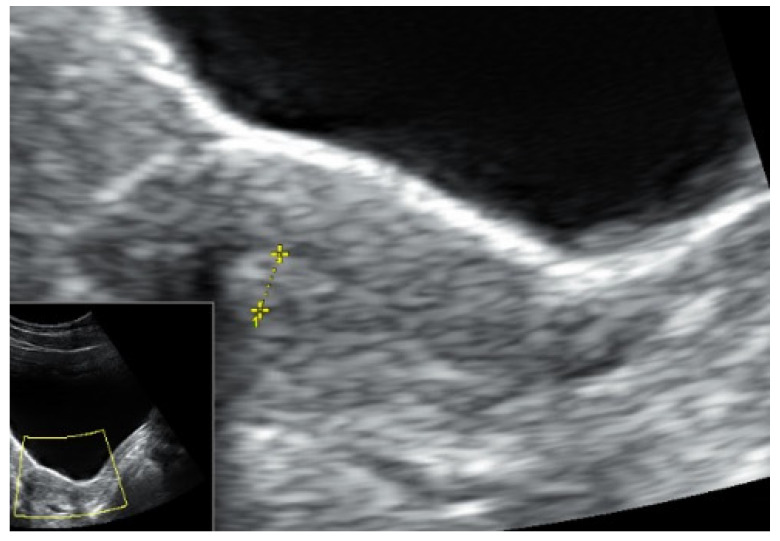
Abdominal sonography of the messurement of the tissue of the uterus in an adolescent girl (photo by the authors).
